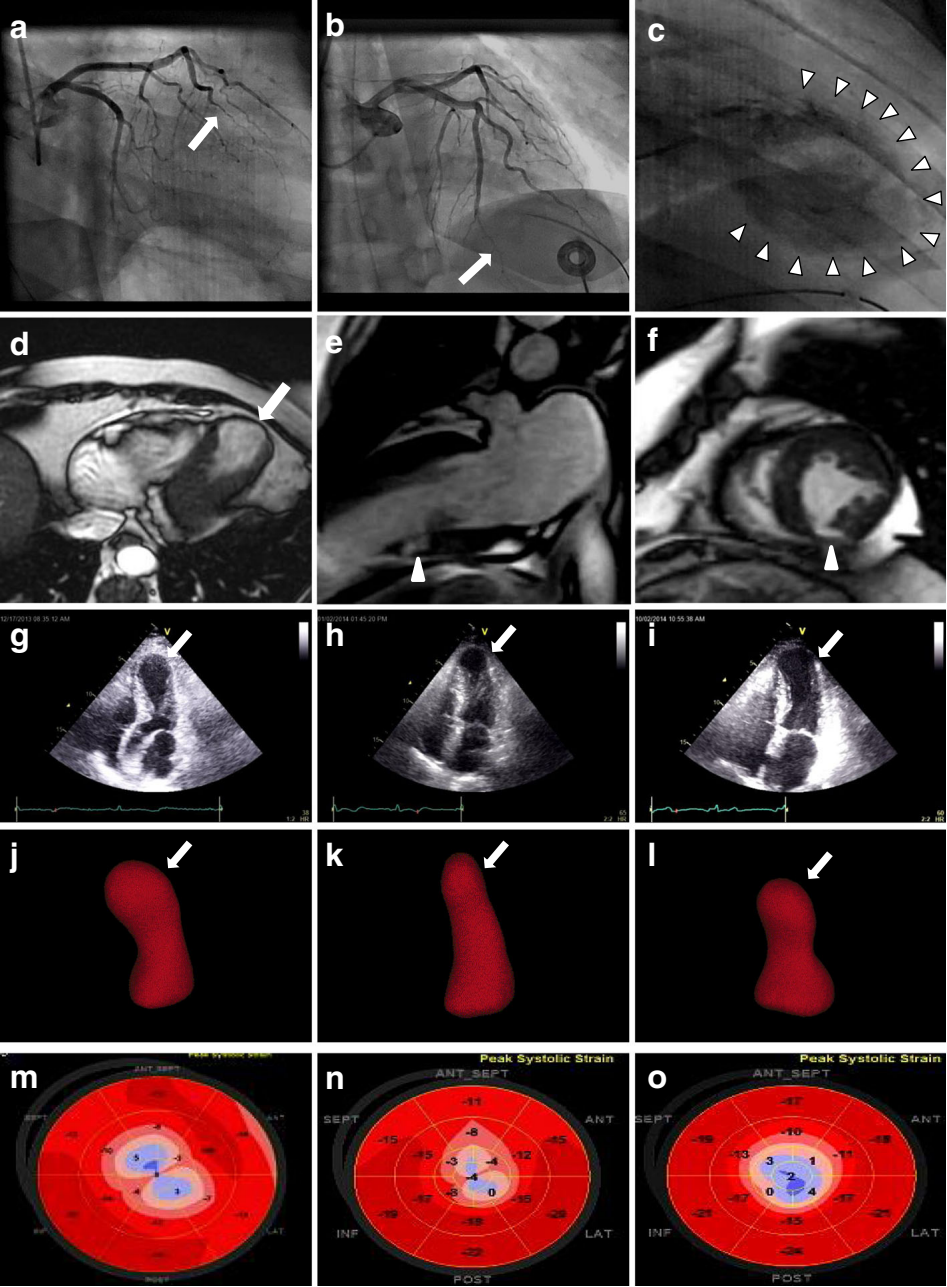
Recurrent Tako-tsubo syndrome (TTS) in a woman with coronary artery fibromuscular dysplasia and left ventricular apical infarction. a (right anterior oblique caudal projection) and (b) (right anterior oblique cranial projection): The coronary angiograms showing a tapering and smooth narrowing (with a discrete transition from normal to diseased artery) in distal left anterior descending artery (arrows) .c The Ventriculography (right anterior oblique projection) demonstrating apical ballooning involving broader myocardium beyond the territory of distal LAD (arrowheads). d Non-contrast cardiac magnetic resonance (CMR) images indicating dilated left ventricle (arrow). e Two chamber view contrast CMR with late gadolinium enhancement (LGE) image revealing localized inferoapical myocardial infarction (arrowhead). f Short-axis view contrast CMR with LGE image revealing localized inferoapical apical myocardial infarction (arrowhead). g-o Recurrent TTS with alternated ventricular contractile patterns. g, h, i Two dimensional echocardiograms; (j, k, l): Three-dimensional echocardiograms; M,N,O: Speckle tracking echocardiograms (automated function imaging with a “bull’s eye” plot). g, j, and m: Initial TTS; (h), (k) and (n): recovery period; (i), (l), and (o): recurrent TTS
